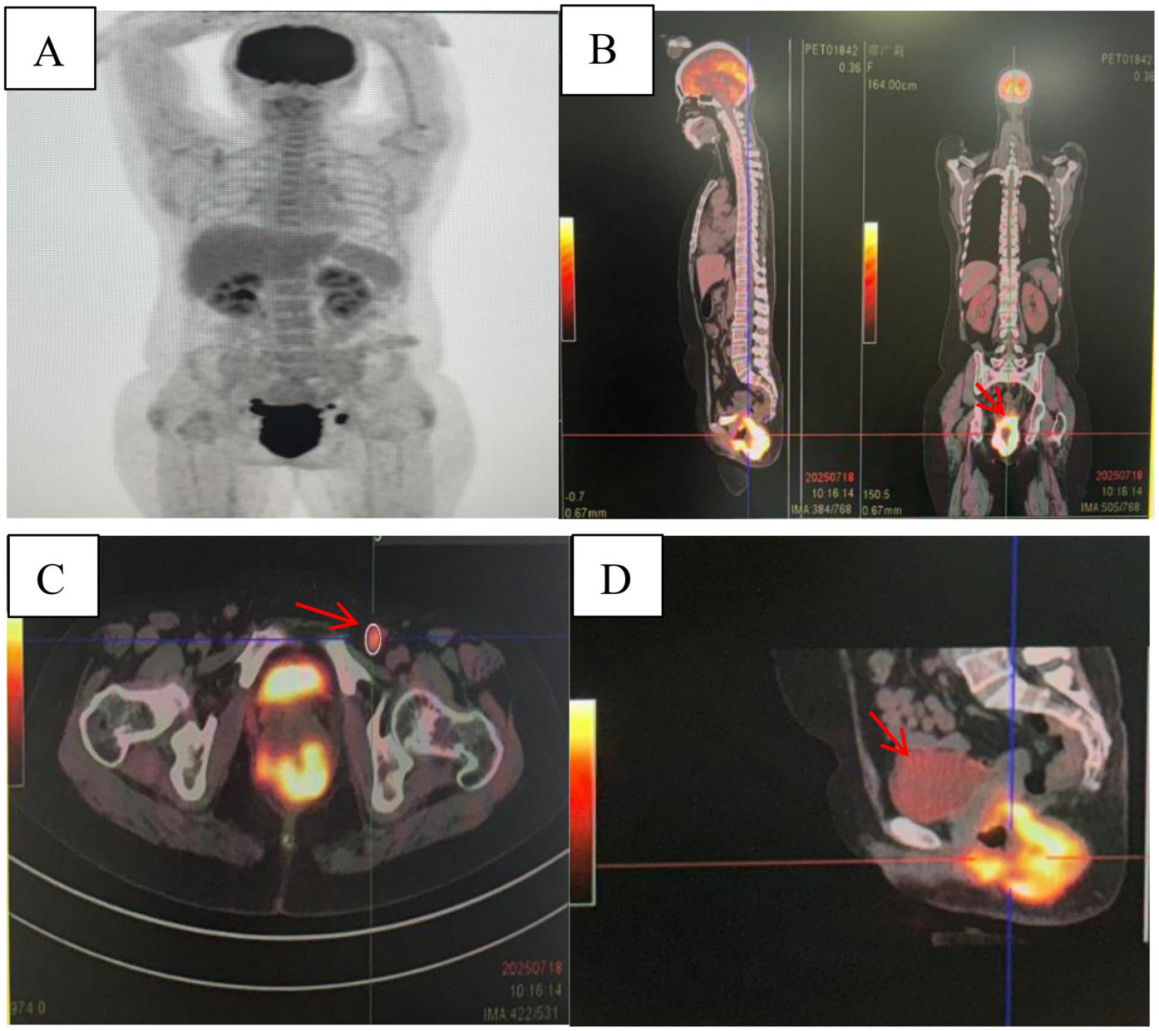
PET-CT: (A) fluorodeoxyglucose (FDG) accumulation was observed in the marked areas (black deposits). (B) Red arrow: Uneven thickening and soft tissue mass formation were seen around the rectum-anal canal, with an unclear boundary with the middle and lower segments of the vagina and abnormally increased FDG uptake. (C) Red arrow: Enlarged inguinal lymph nodes. (D) Red arrow: The bladder wall was not significantly thickened, and no abnormal density shadows were found inside; the distribution of F-FDG uptake was normal.
